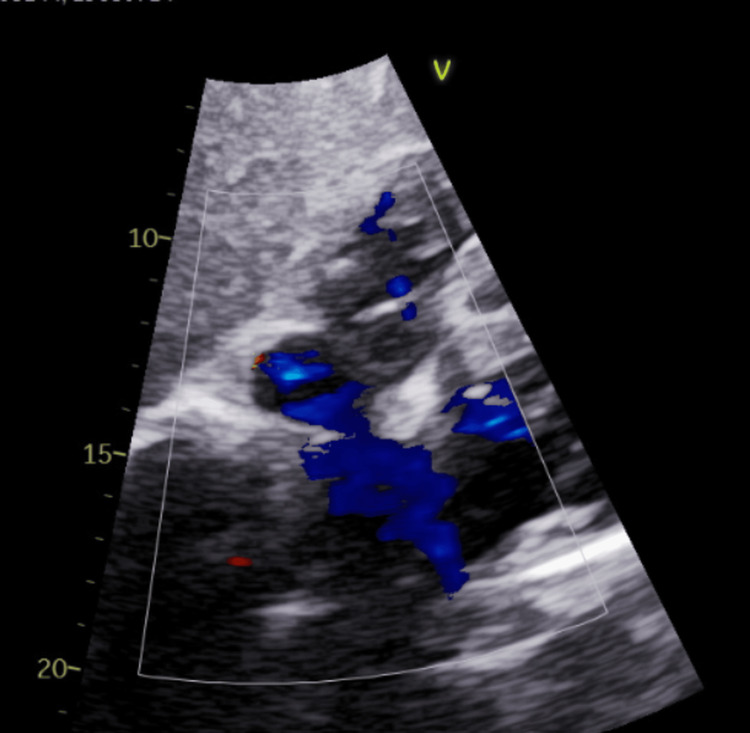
Subcostal Color Doppler Echocardiogram Demonstrating Right-to-Left Shunt Subcostal color Doppler image demonstrating right-to-left interatrial shunting across the atrial septum, with blue color Doppler signal indicating right-to-left flow.
